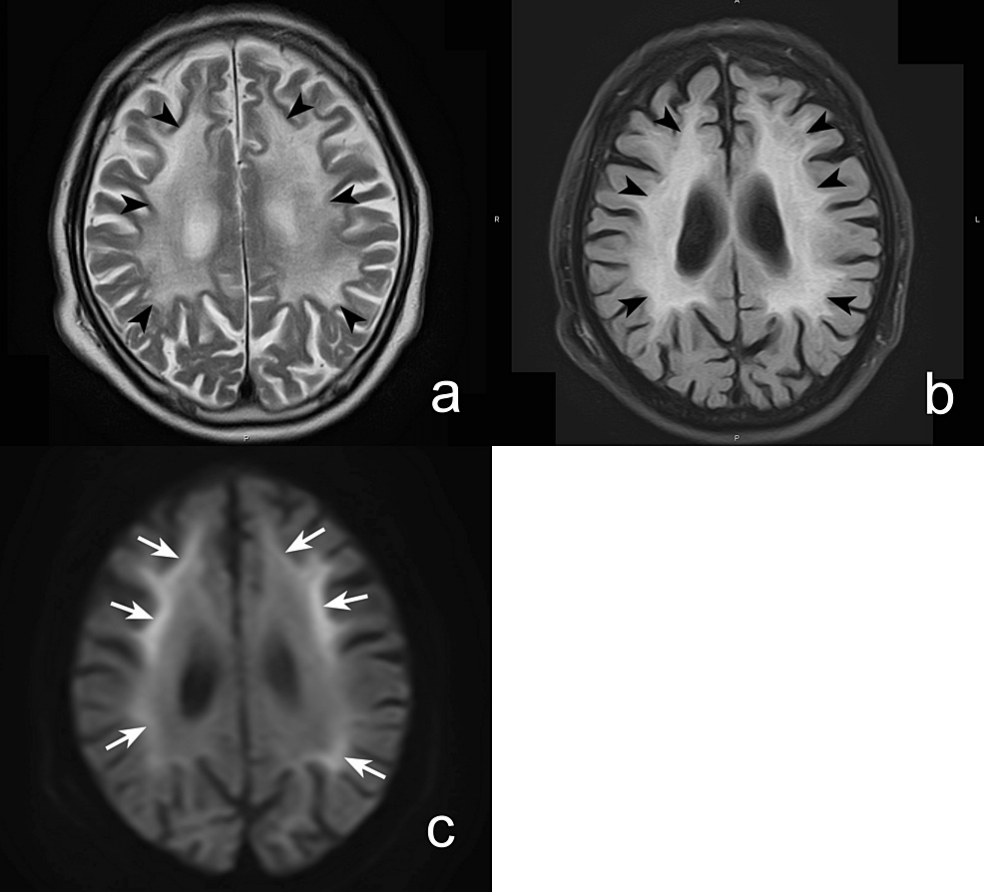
Non-contrast-enhanced head magnetic resonance imaging performed in late October 2021 Diffuse white matter lesions (arrowheads) on T2-weighted (a) and fluid-attenuated inversion recovery images (b), and characteristic high signal at the corticomedullary border (arrows) on diffusion-weighted images (c) were noted.
